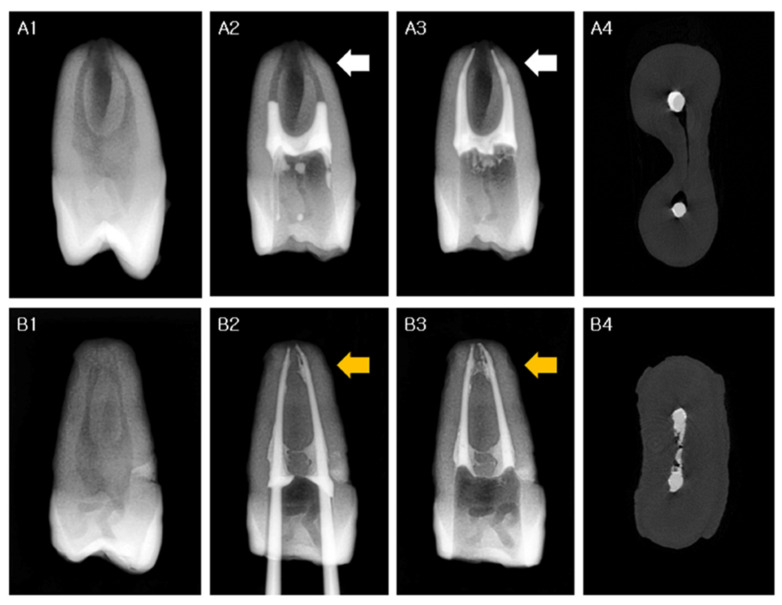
Representative images of root canal sealer movement at the apical thirds of SE group (A1–A4) and VG group (B1–B4). (A1,B1): pre-operative standard radiographic images of each group. (A2–A4): before (A2) and after (A3) single-cone technique + Endoseal TCS; micro-CT cross-section image at the apical thirds (A4), indicated by the white arrow in (A2,A3). Movement of Endoseal TCS into the complex apical anatomy can barely be noticed. (B2–B4): before (B2) and after (B3) the VibraTHO technique + GuttaFlow 2; micro-CT cross-section image at the apical thirds (B4), indicated by the yellow arrow in (B2,B3). Even though it is not perfectly filled, hydrodynamic movement of GuttaFlow 2 into the complex apical anatomy after application of the VibraTHO technique is clearly observed. CT: computed tomography, VibraTHO: ultrasonic vibration and thermo-hydrodynamic obturation.
